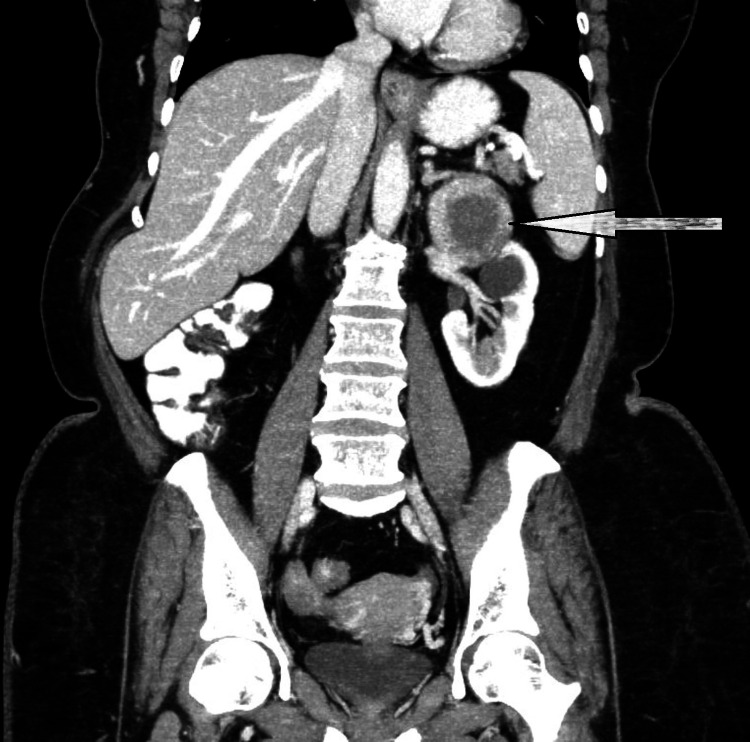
Coronal CT of abdomen and pelvis A large well-defined hypoattenuating, non-enhancing cystic lesion of size 71 x 61 x 56 mm, surrounded by enhancing adrenal tissue was noted arising from the inferolateral aspect of the left adrenal gland, seen abutting the tail of the pancreas and the small bowel loop (arrow). It showed no solid components/septations/calcifications.
